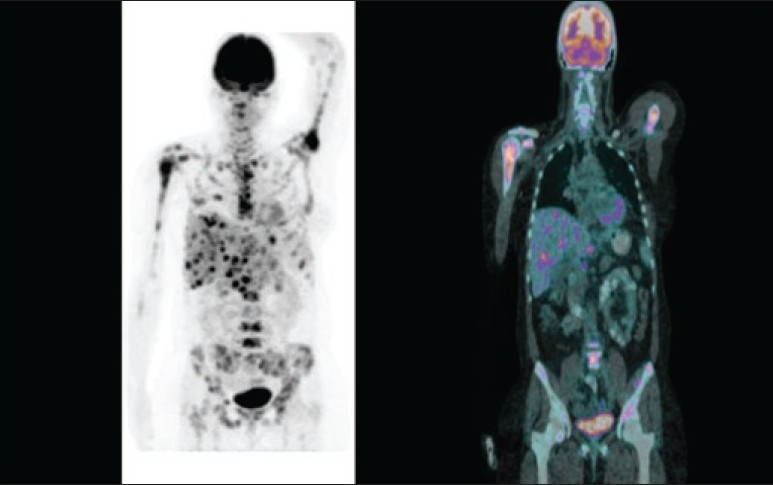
A 66-year-old woman who came for restaging of breast cancer. MIP image (left panel) and coronal fused images (right panel) of 18F-FDG PET/CT showed extensive hepatic and bony metastases.
